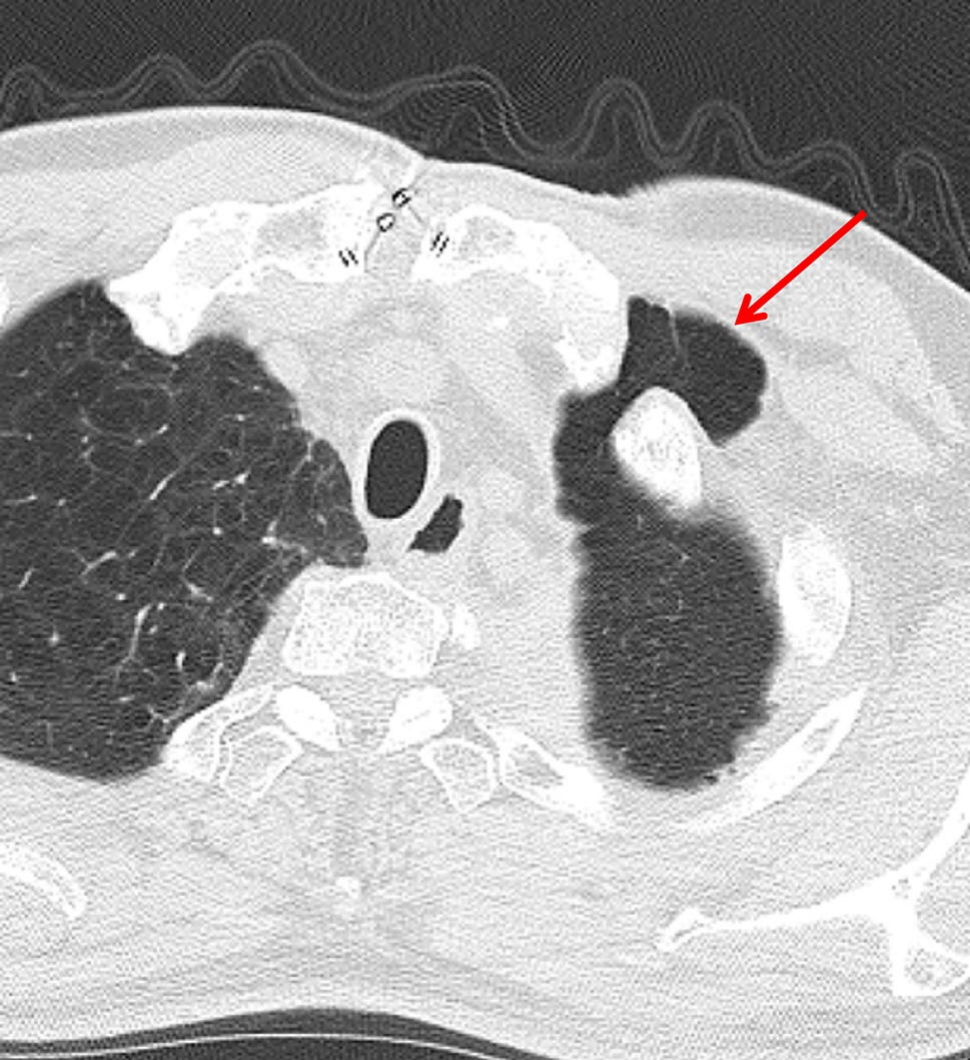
Postoperative lung hernia. Two years after left upper partial lobectomy, CT demonstrates lung parenchyma protruding through a chest wall defect at the left lung apex
